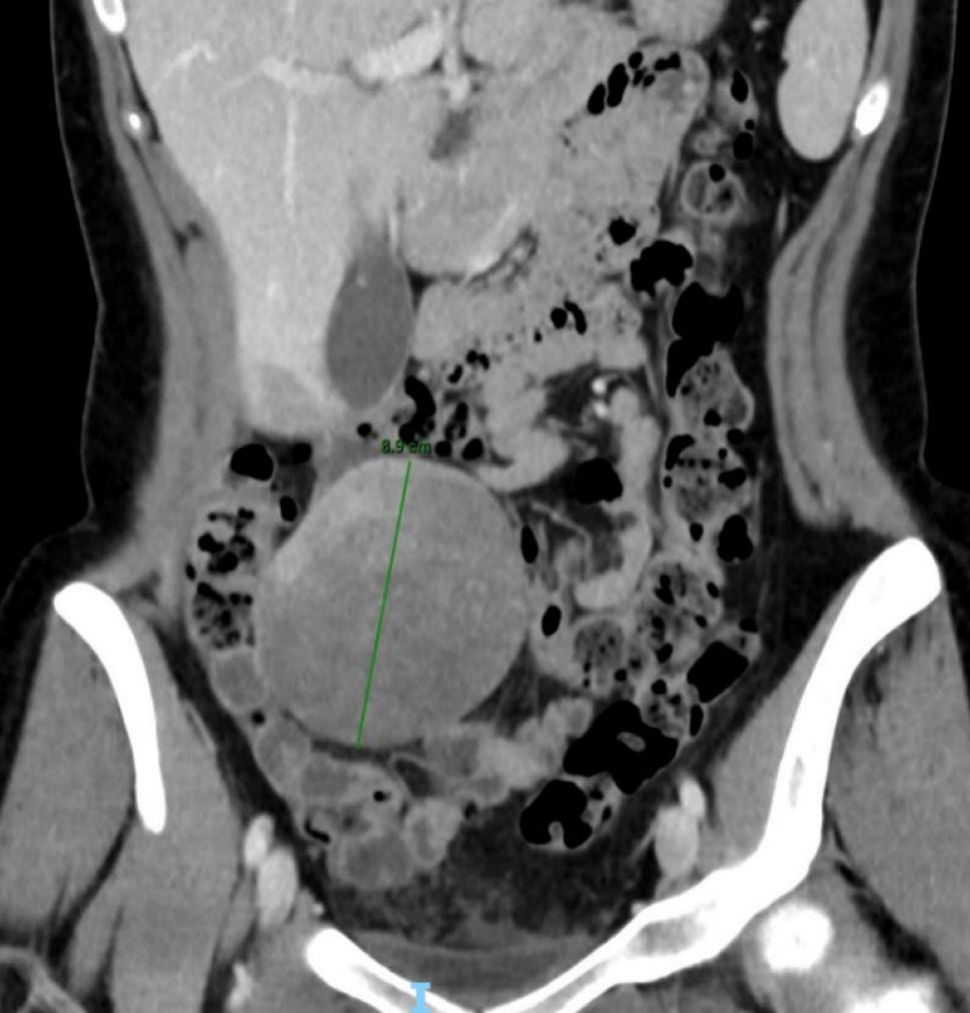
Abdominal CT scan with coronal reconstruction showed a solid formation with well-defined margins, measuring 86 × 65 × 88 mm, with progressive, not homogeneous late contrast enhancement, especially at the superior pole
